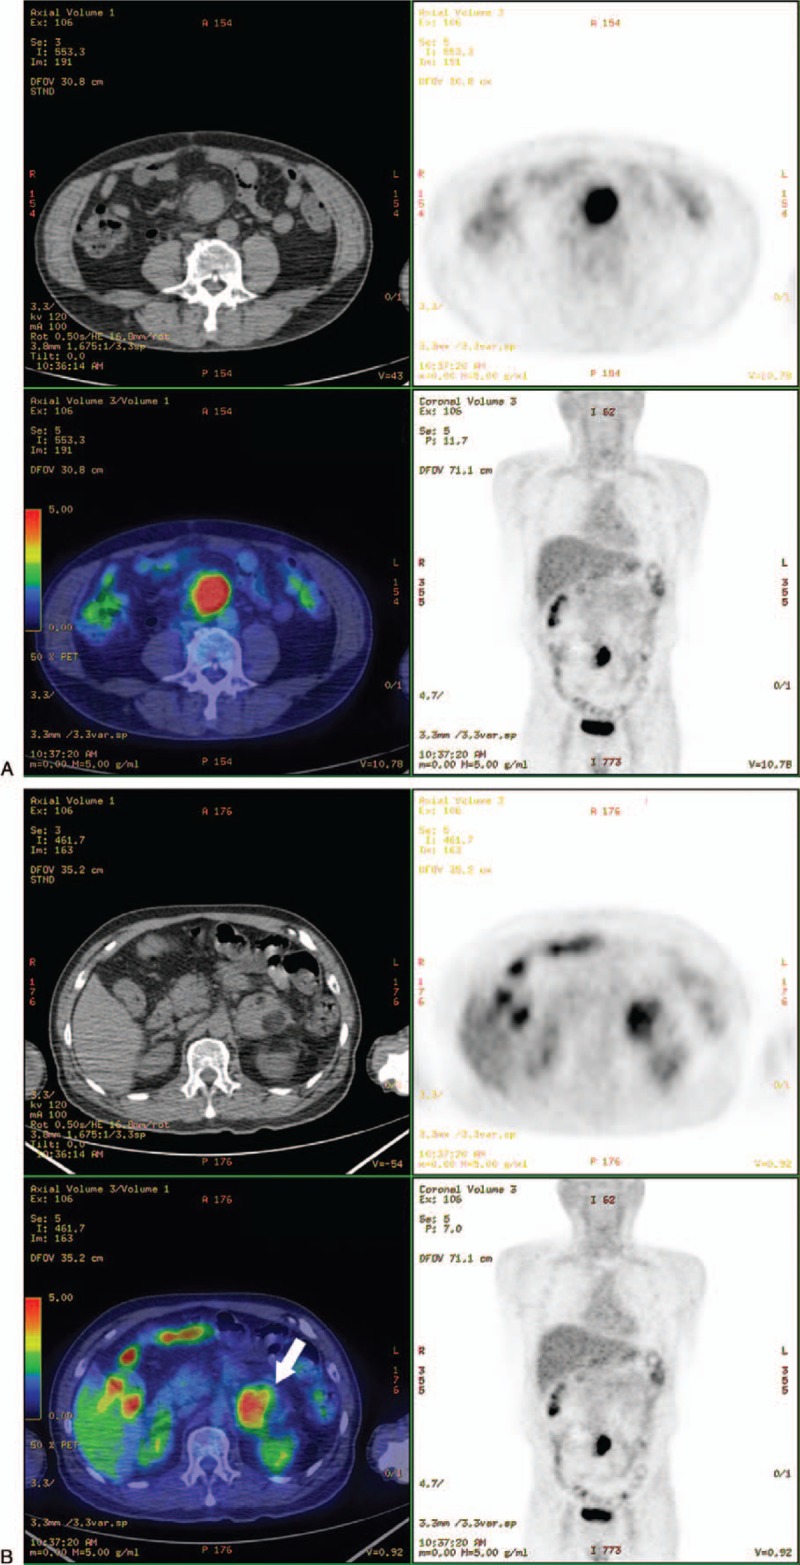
Positron emission tomography-computed tomography showing (A) A hypermetabolic mass in the mesentery suggesting primary mesenteric tumor (peak standardized uptake value [SUV] = 11.8); (B) A hypermetabolic mass in the left adrenal gland suggesting benign adrenal gland tumor (peak SUV = 5.5) (arrow).
